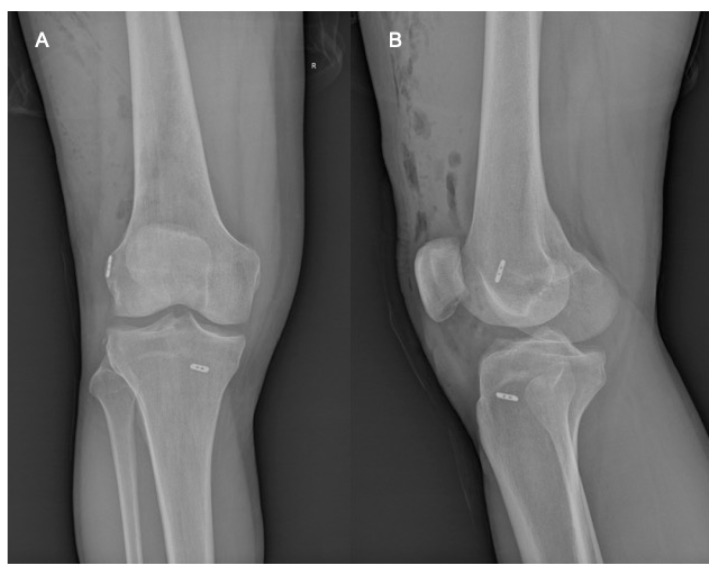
Post-operative X-rays, Aanteroposterior (A) and lateral (B).
